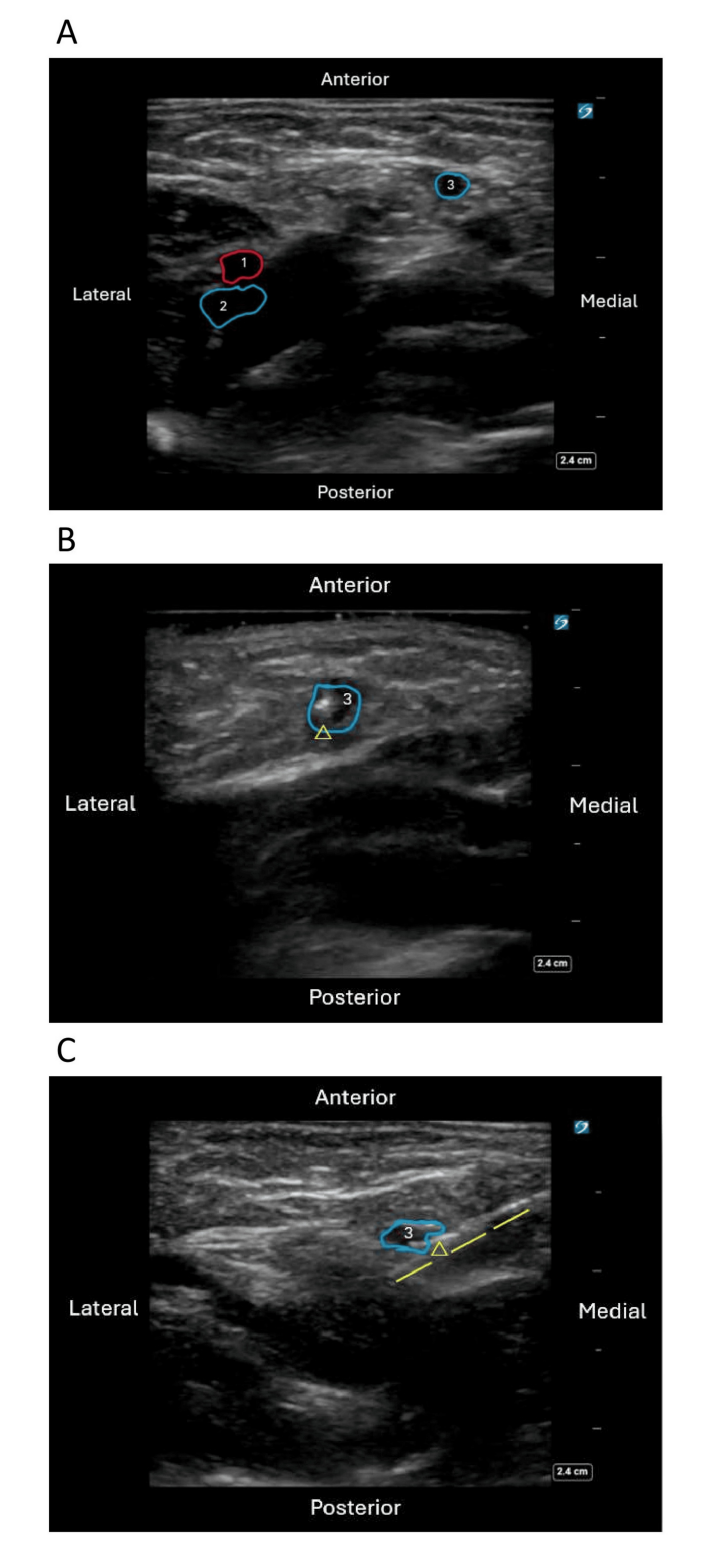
Ultrasound confirmation of needle tip location (A) Redemonstration of the anatomy of the GS vein in relation to the femoral artery and vein in the axial plane. (B) Needle tip in the GS vein perpendicular to the ultrasound probe in the axial plane. (C) Sagittal plane view of the needle tip in the GS vein. 1: femoral artery; 2: femoral vein; 3: GS vein GS: greater saphenous
